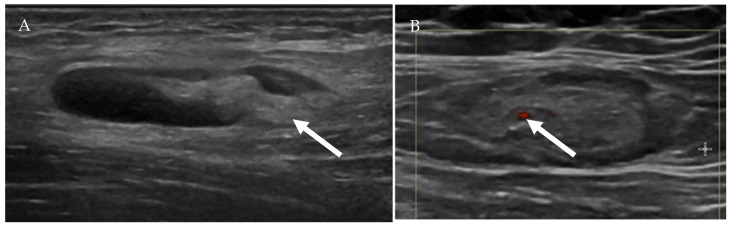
Ultrasound of a benign-appearing cervical lymph node (A) shows an oval hypoechoic lymph node with echogenic fatty hilum (arrow). Color doppler ultrasound of another benign-appearing lymph node (B) demonstrates a fatty hilum (normal) with mild hilar vascularity (arrow).
